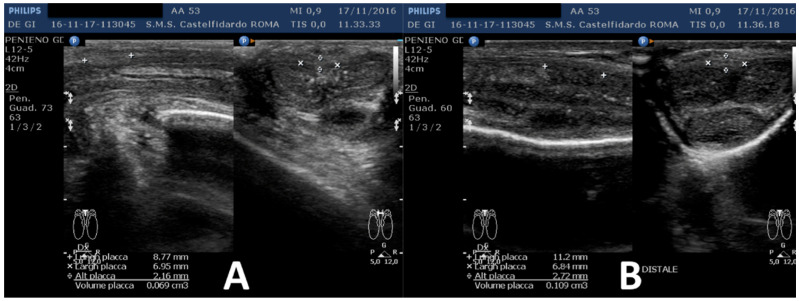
Ultrasonography of the penis after the second treatment cycle. (A) First plaque, (B) second plaque.
